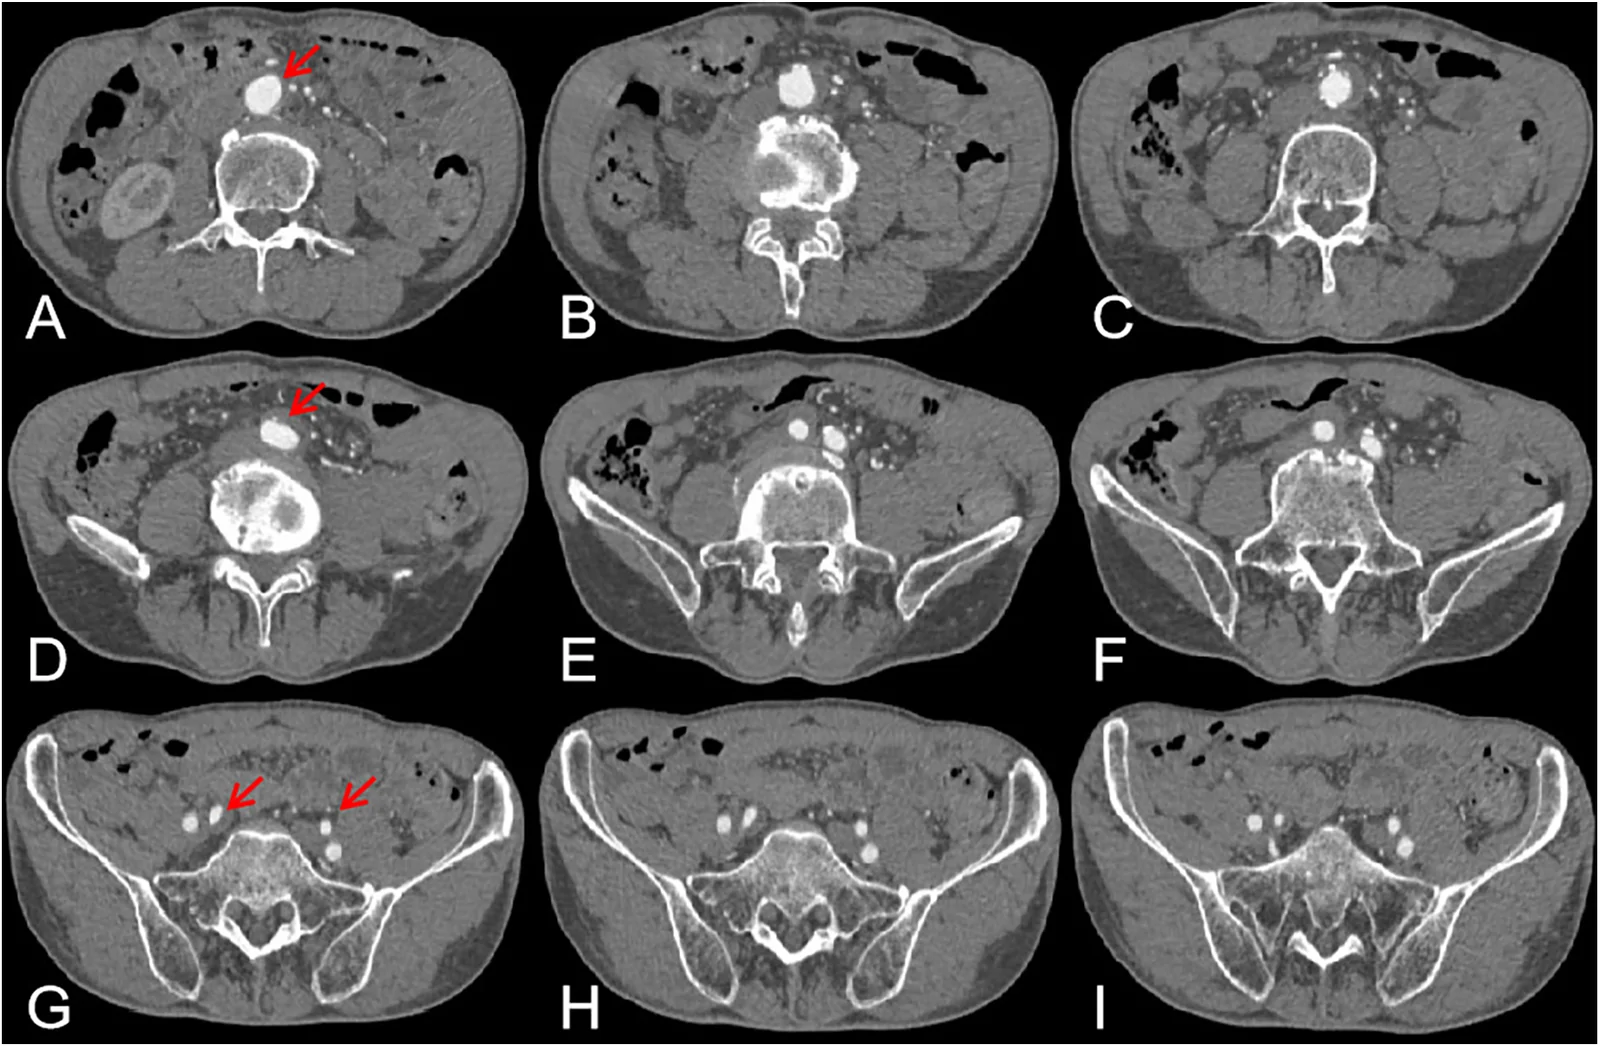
Follow-up CTA after initial treatment (A–C) distal abdominal aorta level: the dissection flap (arrow) persists with stable intramural hematoma. (D–F) Aortoiliac bifurcation level: The dissection (arrow) remains unchanged, showing no interval progression. (G–I) Iliac artery level: The dissection in the iliac arteries (arrows) is stable, with reduced hematoma density indicating early organization.
